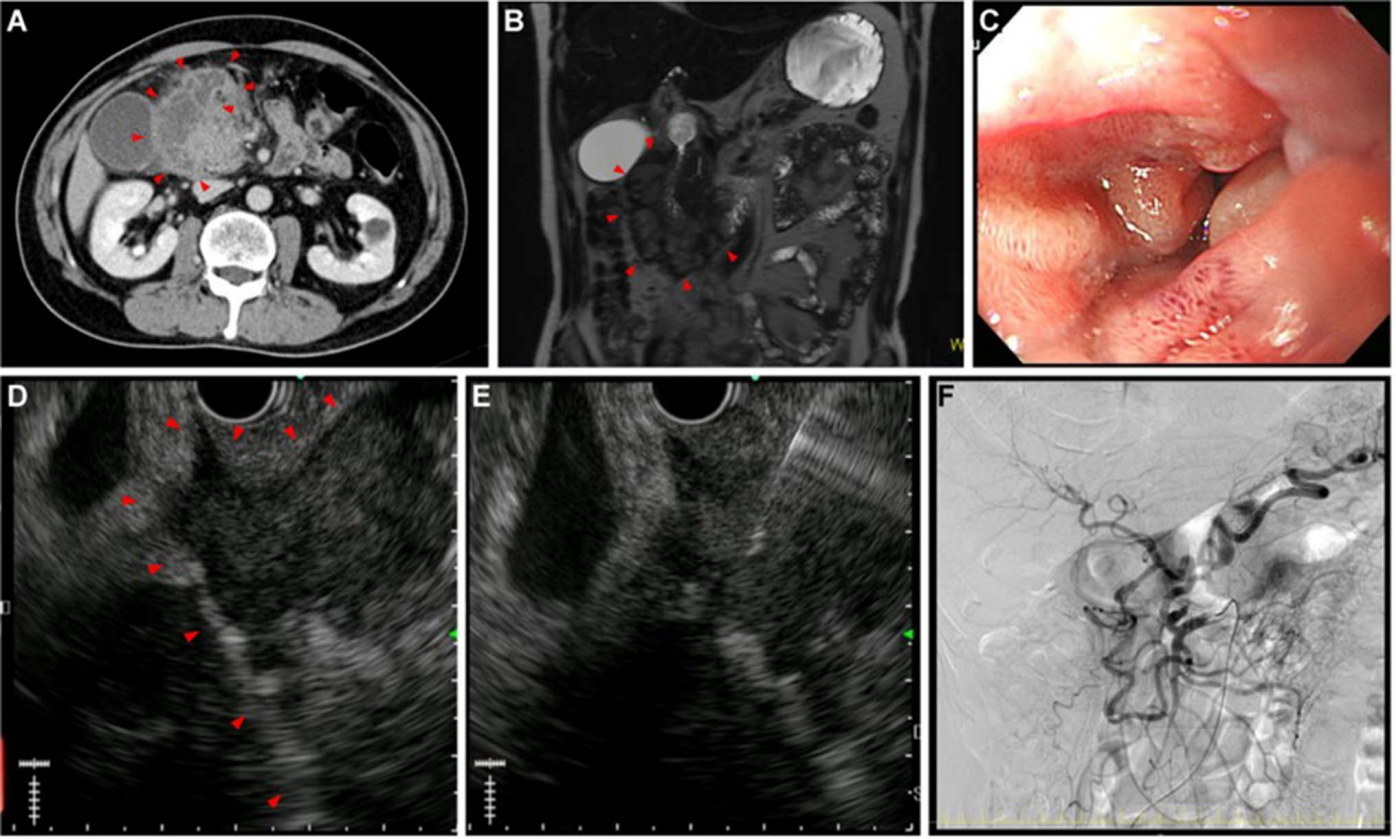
A 64-year-old male presenting with abdominal fullness and vomiting. a Abdominal contrast-enhanced CT on day 2 revealed a 9-cm-sized, low-density mass (arrowheads) around the 2nd to 3rd part of the duodenum. The capsule and internal septum of the mass were enhanced. b Abdominal contrast-enhanced magnetic resonance imaging on day 3. On T2-weighted image, the rim of the mass each revealed a weak signal and the inside of the mass revealed a middle signal (arrowheads). c EGD on day 6 showed edematous stenosis of the 2nd portion of the duodenum. d EUS on day 9 revealed a large cystic mass around the duodenum (arrowheads). The mass showed low echogenicity with a partially anechoic area and a debris-like area of slightly high echogenicity, and these were not obvious findings of a malignant tumor. There was no apparent vessel structure in the mass. e Fine-needle biopsy was performed to assess the possibility of infection. The pathological result was the existence of peripheral blood with a small amount of fibrous tissue and glandular epithelium. No bacteria were identified in the culture test. f An angiography on day 15. Selective angiography of the superior mesenteric artery (SMA) showed a remarkably dense network of collateral vessels connecting the SMA to the celiac artery. There was an irregular caliber change in the anterior pancreaticoduodenal arcade. Since there were no apparent findings of aneurysms or arteriovenous malformation, endovascular embolization was not performed
